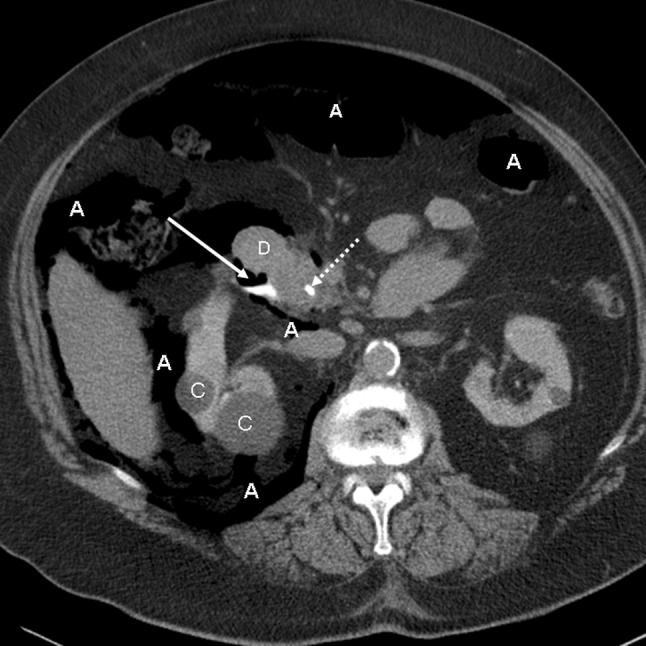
Case 1* Abdominal CT-scan with oral contrast administration. At the site of the papilla of Vater there is minimal extravasation of contrast indicating perforation (*solid arrow*). *Dotted arrow* biliary endoprosthesis in duodenum, *A* intra- and retroperitoneal air, *C* renal cortical cysts, *D* duodenum
